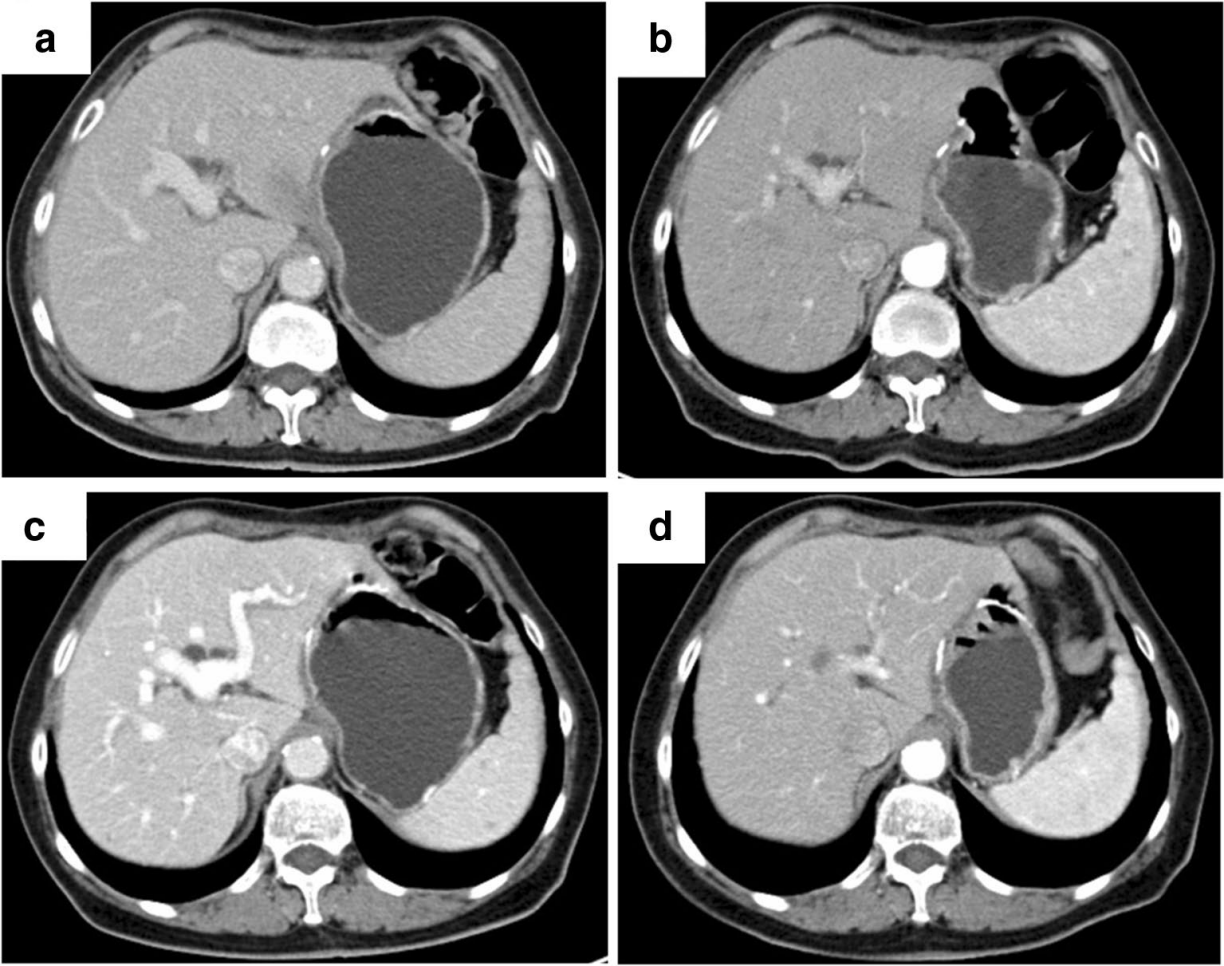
Abdominal computed tomography scans 6- (a), 12- (b), 18- (c), and 24- (d) months after surgical therapy. The scans do not exhibit any recurrence of the tumor or metastatic lymph nodes
